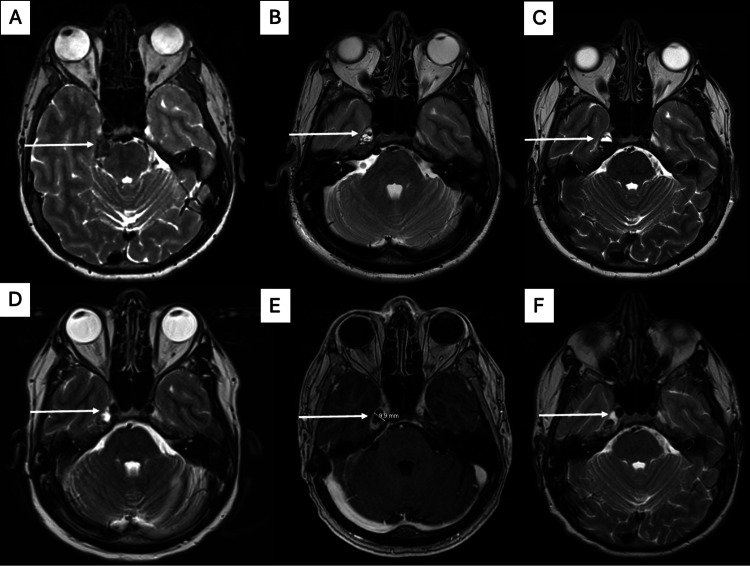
Right Meckel’s cave malignant melanotic nerve sheath tumor (Case 2). Axial T2-weighted MR images, with arrows indicating the tumor, demonstrating (A) preoperative tumor extent, (B) postoperative residual tumor, (C) tumor extent immediately prior to SRS, (D) the irradiated tumor at the first follow-up, 1.6 months after SRS, (E) increased cystic change within the tumor, representing post-treatment tumoral swelling, 10 months after SRS, and (F) stability of the treated tumor, 1.6 years after SRS.
